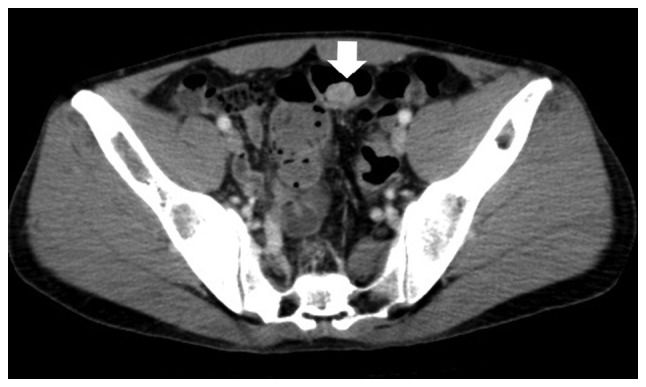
Preoperative contrast-enhanced computed tomographic scan of the abdomen. The arrow indicates an enhanced mass (15×14 mm) in the rectum.
